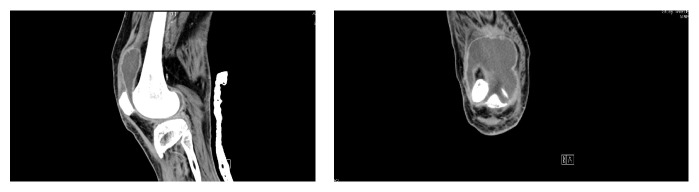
CT scan with moderate to large joint effusion of the (R) knee with associated synovial thickening and OA.
